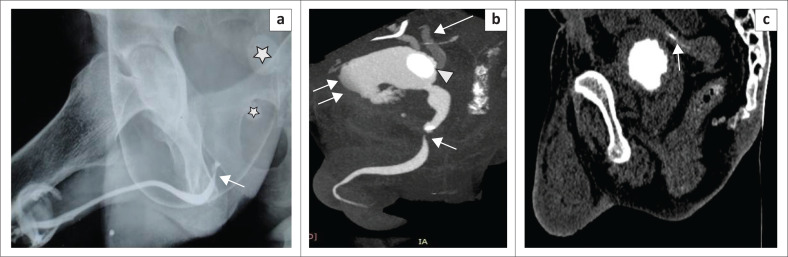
(a) Retrograde urethrography (RGU) image showing a normal anterior urethra with tapering at the bulbomembranous junction (arrow) and no distal passage of contrast into the posterior urethra. Micturating cystourethrography could not be performed because of unsuccessful catheterisation. A confident diagnosis of a stricture could not be made. Two calculi (asterisks) were noted, but exact localisation was difficult on RGU. (b) Sagittal maximum intensity projection image (multidetector computed tomographic urethrography [MDCTU]) demonstrates the membranous urethral stricture, posterior urethral calculus (short arrow), vesical calculus (arrowhead), irregular shaped bladder with contrast leakage into the perivesical tissues (double arrows) and bilateral vesico-ureteric reflux (VUR) (long arrow). (c) Sagittal multiplanar reformatting image (MDCTU) of the same patient showing part of the rectum in close proximity to the dome of the bladder, with loss of intervening fat planes and contrast noted inside the rectum (arrow), suggestive of rectovesical fistula.
